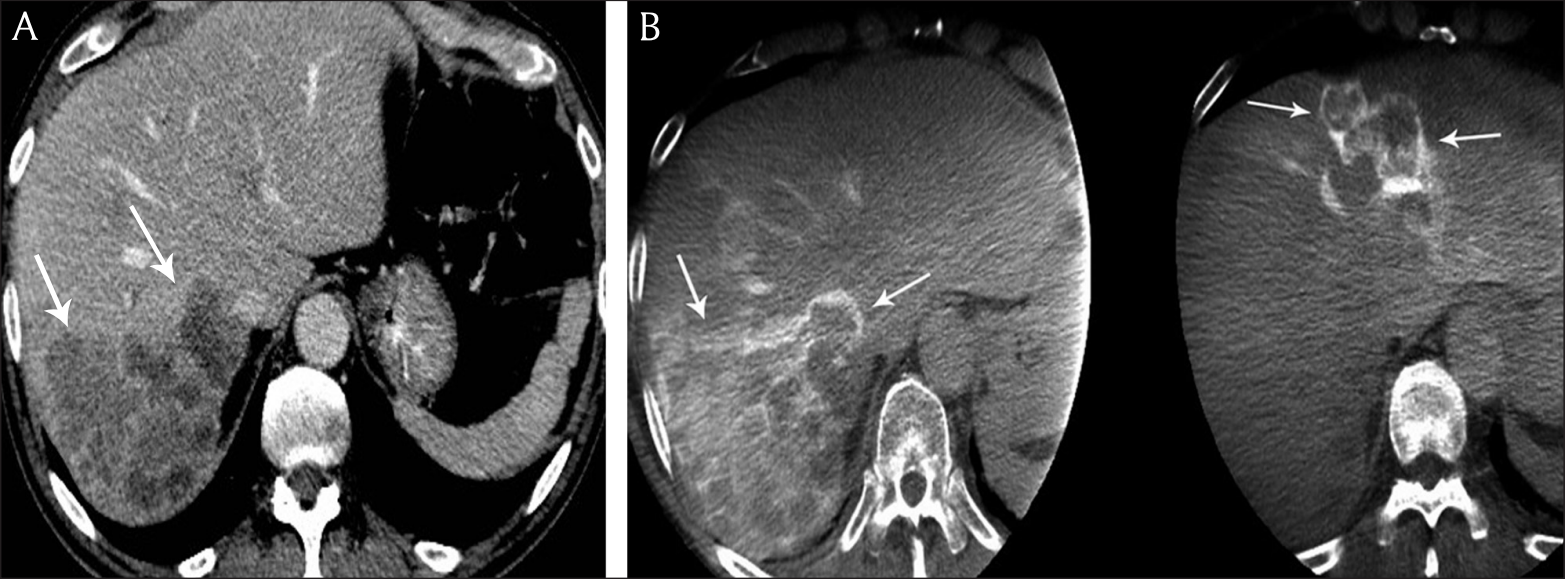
A) Contrast-enhanced computed tomography and B) cone beam computed tomography in a 68-year-old man presenting with bilobar colon cancer liver metastases. Contrast-enhancement of the peripheral tumoral rim is hyperdense to the residual liver parenchyma on cone beam computed tomography versus contrast-enhanced computed tomography.
